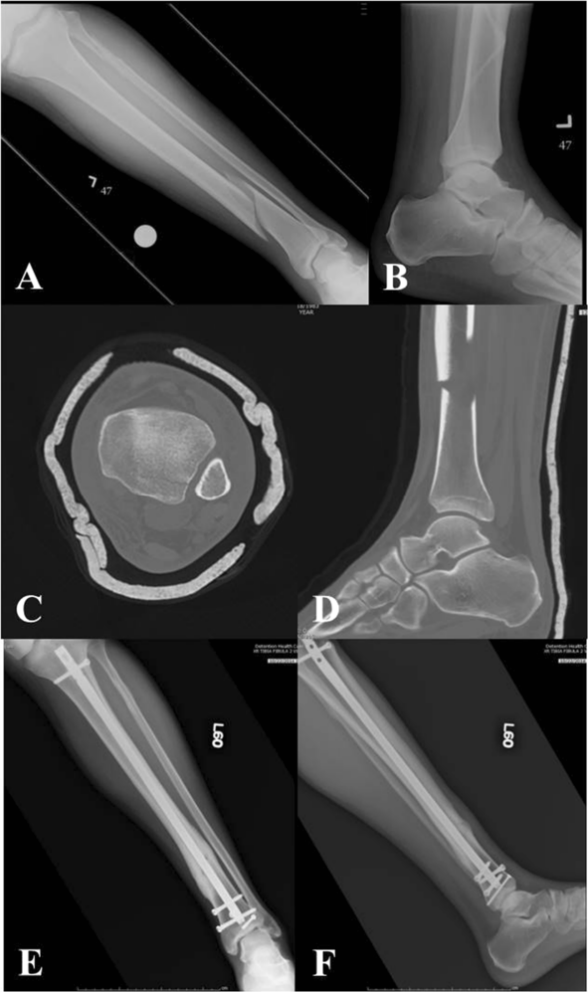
a-f Left distal third spiral tibia fracture (a) with normal appearing radiographs of the ankle (b). Preoperative CT scan shows a non-contiguous minimally displaced posterior malleolus fracture (c-d). Follow-up radiographs (e-f) show uneventful healing of both tibia and ankle injury
